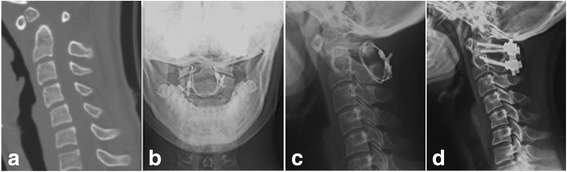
A 17-year-old woman had Os odontoideum. a Preoperative CT showed uncombined odontoid process. b, c Postoperative X-ray obtained at the 6th month after the Gallie fixation showed that there was no bridging bone around the iliac bone graft. d Lateral radiographs obtained in the 24th month after the Gallie fixation showing bone fusion
